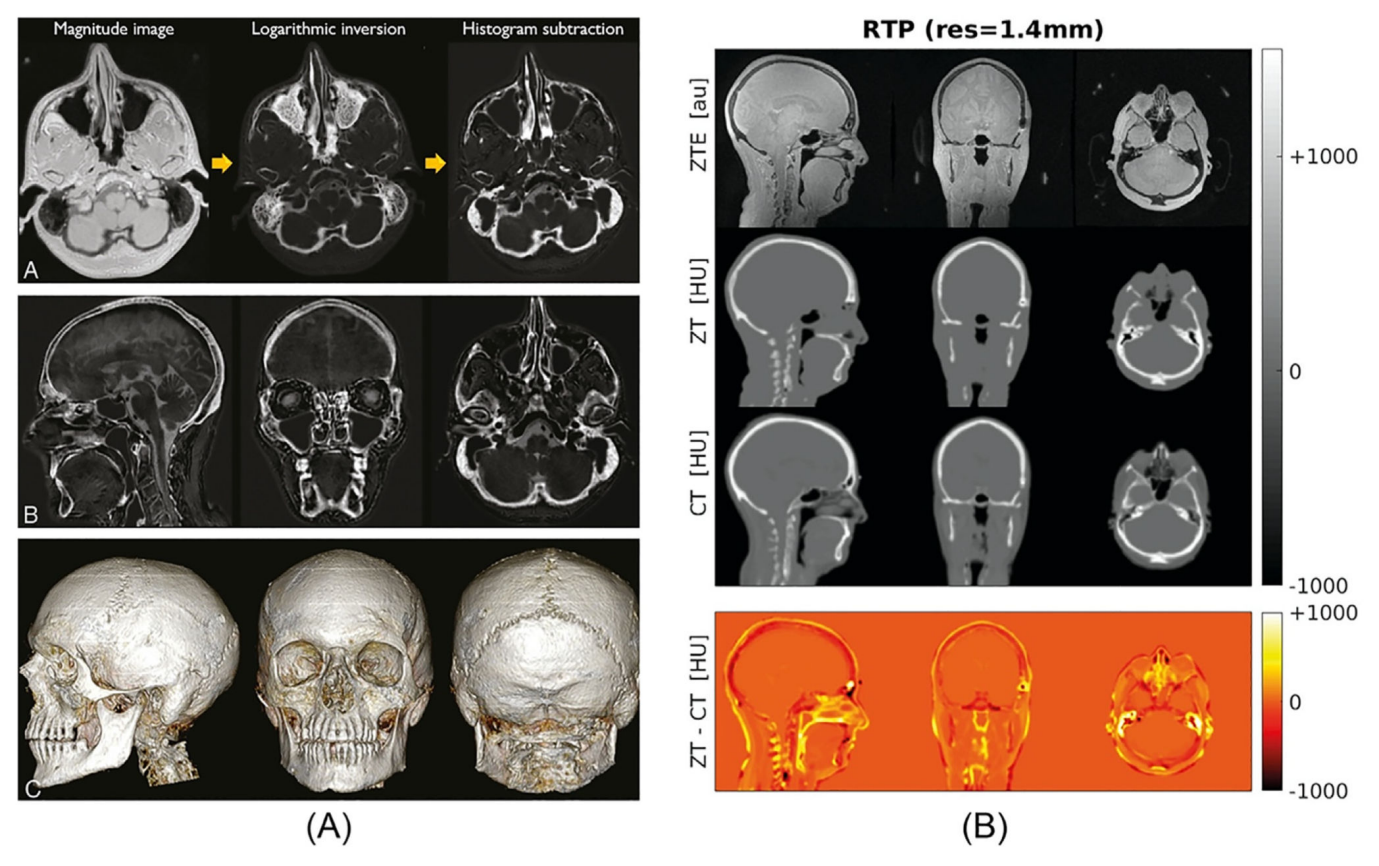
Ultra-short T_2_ PD imaging with ZTE. (A) Skull segmentation. Republished with permission of the American Society of Neuroradiology from Ref. [146]; permission conveyed through Copyright Clearance Center, Inc. (B) Generation of pseudo CT images from ZTE in Hounsfield units, compared to acquired CT (Abbreviations: ZT – ZTE-derived pseudo-CT, RTP – Radiation Therapy Planning, Res – Resolution, HU – Hounsfield Units). Reproduced with permission of John Wiley and Sons from Ref. [147], © 2018 International Society for Magnetic Resonance in Medicine.
